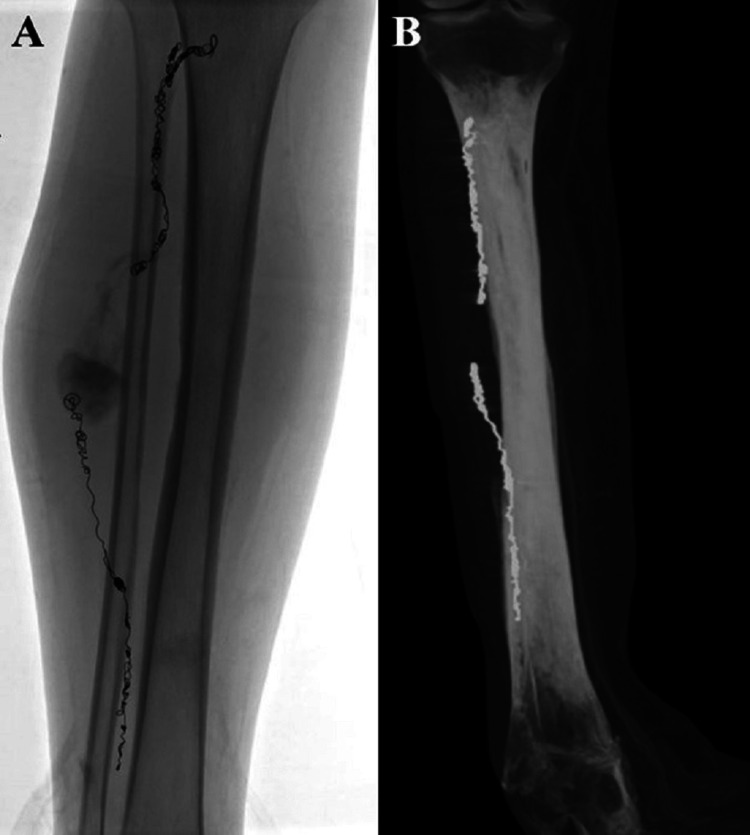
Post embolization and at eight months follow-up A. Spot fluoroscopic image showing endovascular embolization in proximal and distal anterior tibial artery, as well as stagnant contrast in the aneurysm sac. B. CT angiography performed eight months after intervention showing no contrast filling of the pseudoaneurysm.
